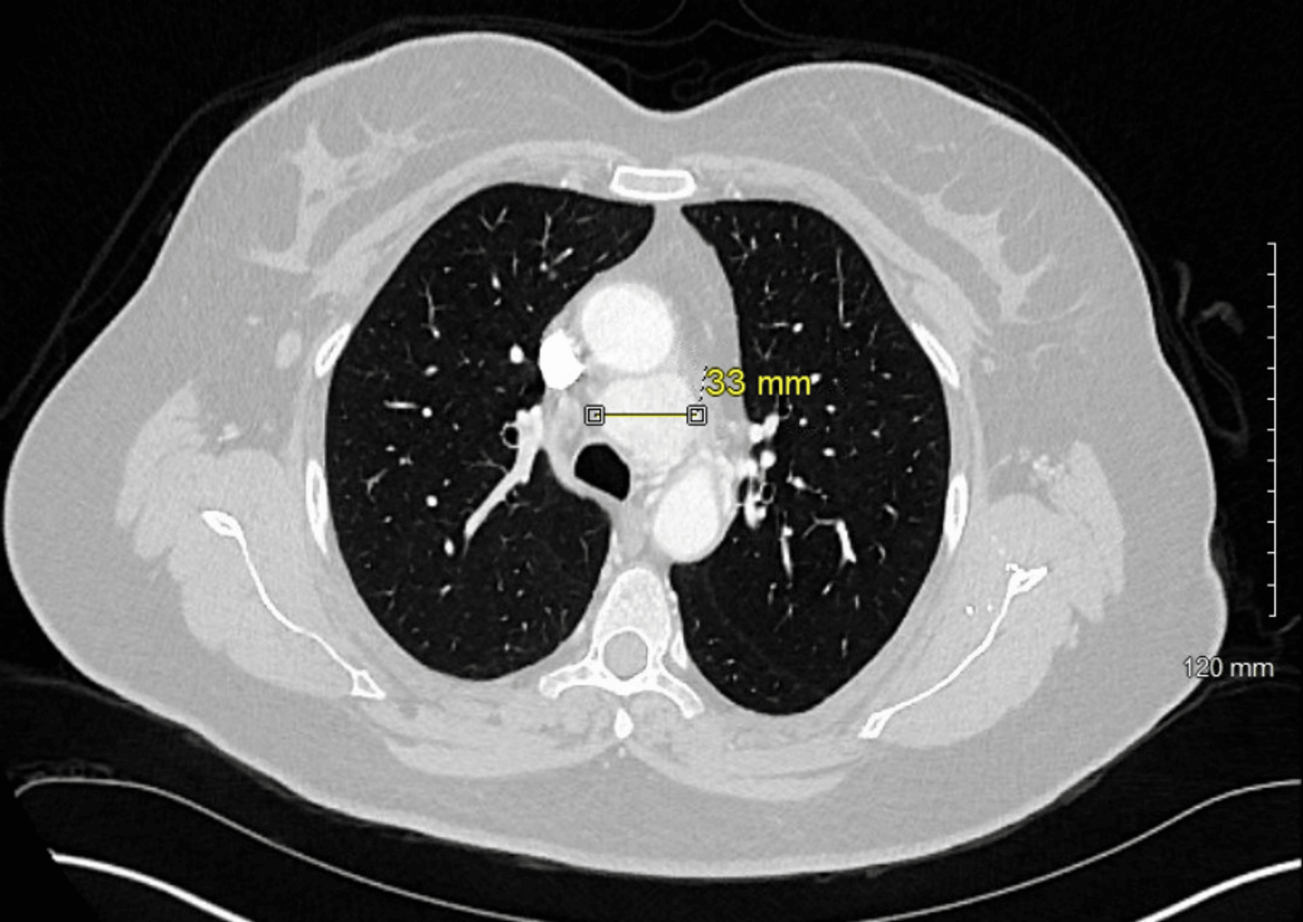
Axial view from CT chest with contrast showing a mediastinal mass with intense and diffuse contrast enhancement
